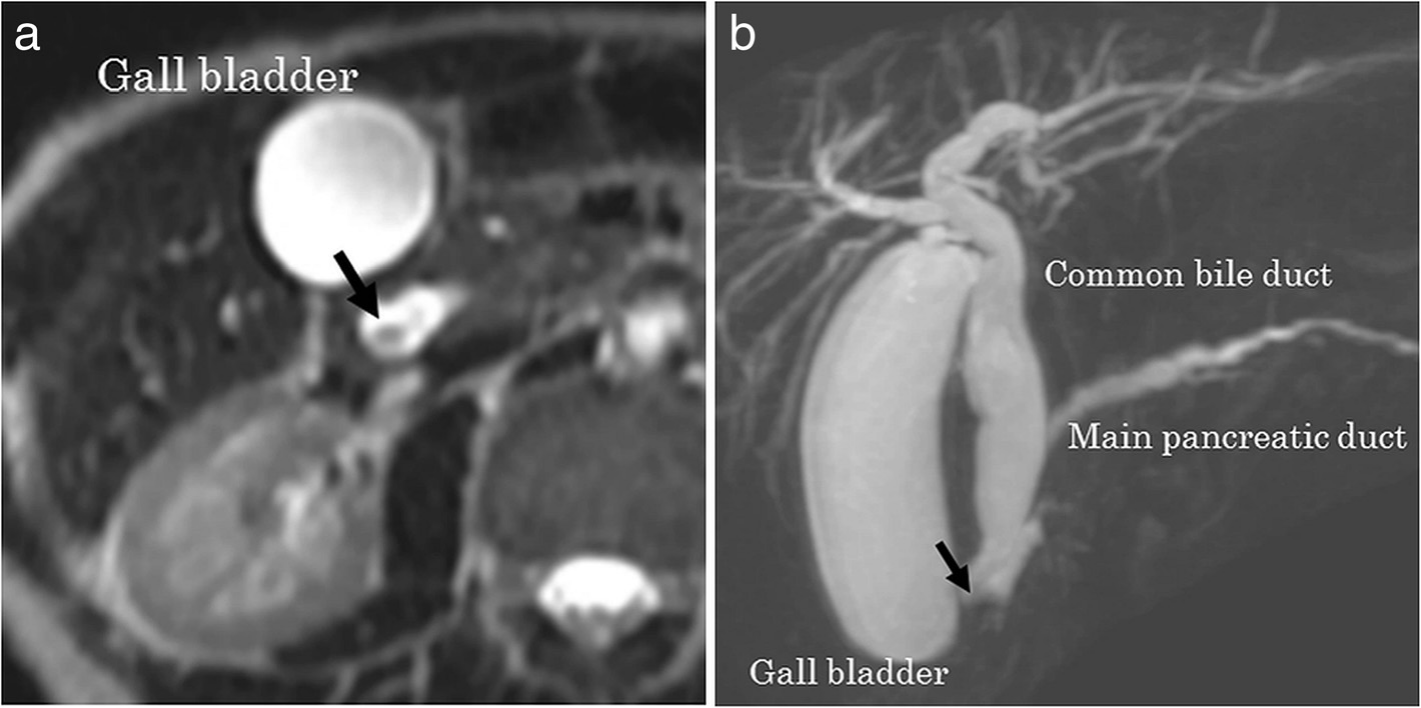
An MRI and MRCP showed the tumor as a defect from the papilla of Vater to the lower CBD (*arrow*) (a, b)
